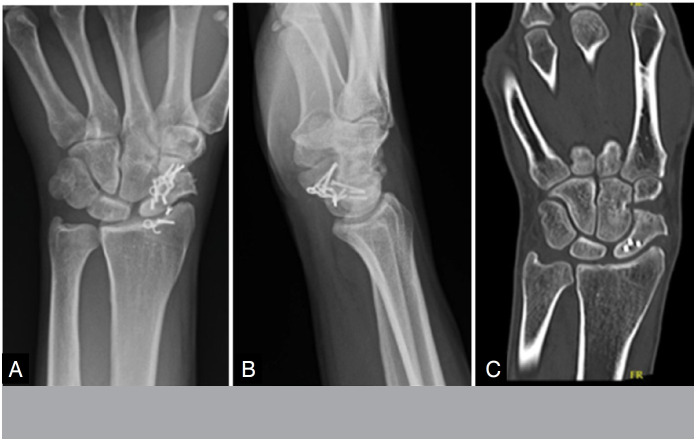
X-rays showing plaque C fracture: tomography with evidence of bone consolidation in the case of plaque fracture.
